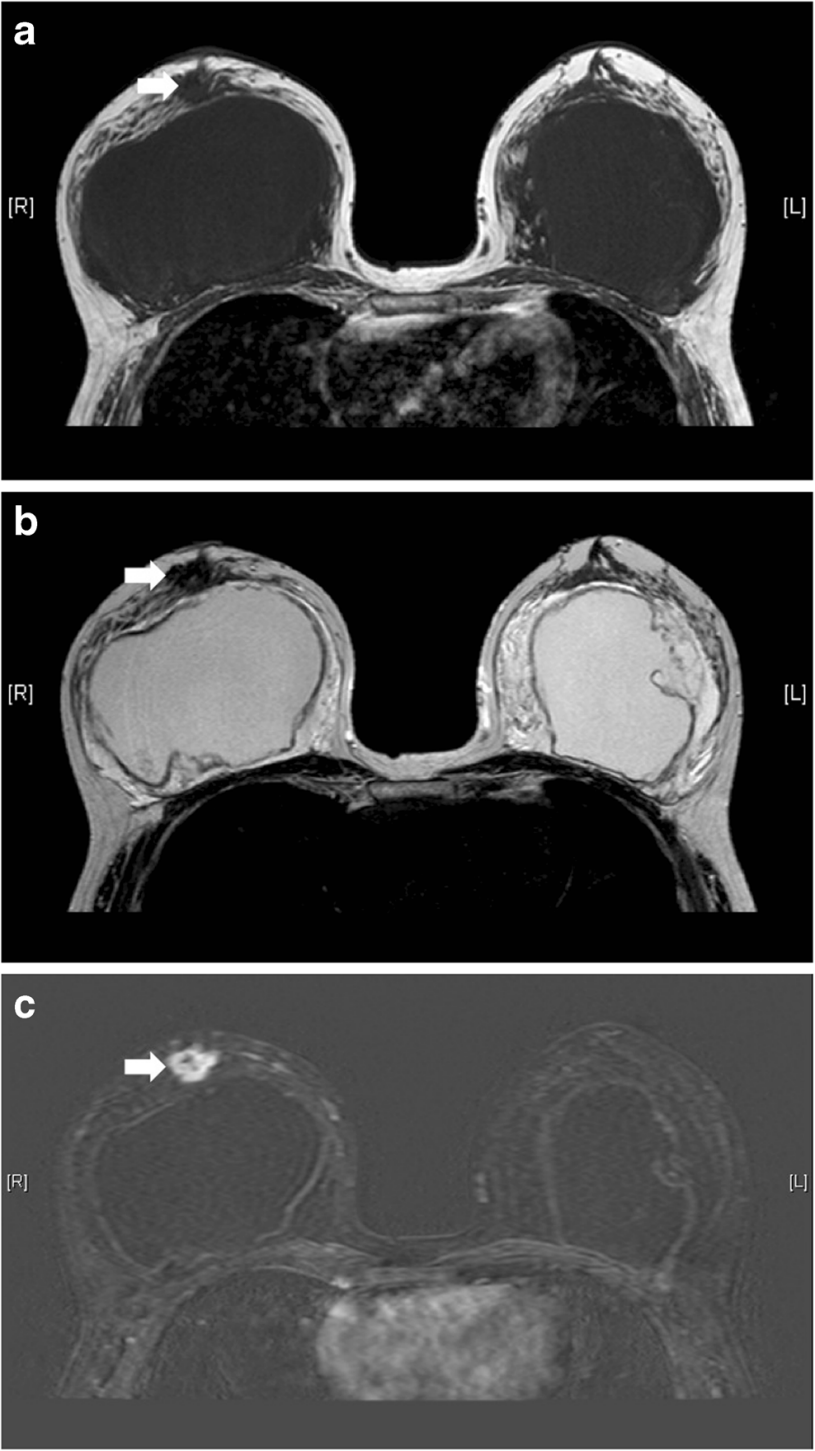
Concurrent breast tumour anterior to right breast implant. T1-weighted (a), T2-weighted (b), and T1-weighted, post-contrast with subtraction (c) axial MR images show a T1 and T2 hypointense lesion with contrast enhancement (arrow) anterior to right breast implant. It was pathologically proven to be invasive ductal carcinoma
